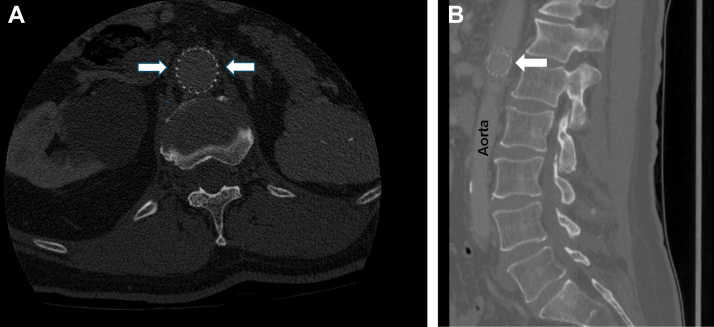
Coronal (A) and sagittal (B) computed tomography images demonstrating the presence of a WATCHMAN device (arrows) within the abdominal aorta (labeled).
